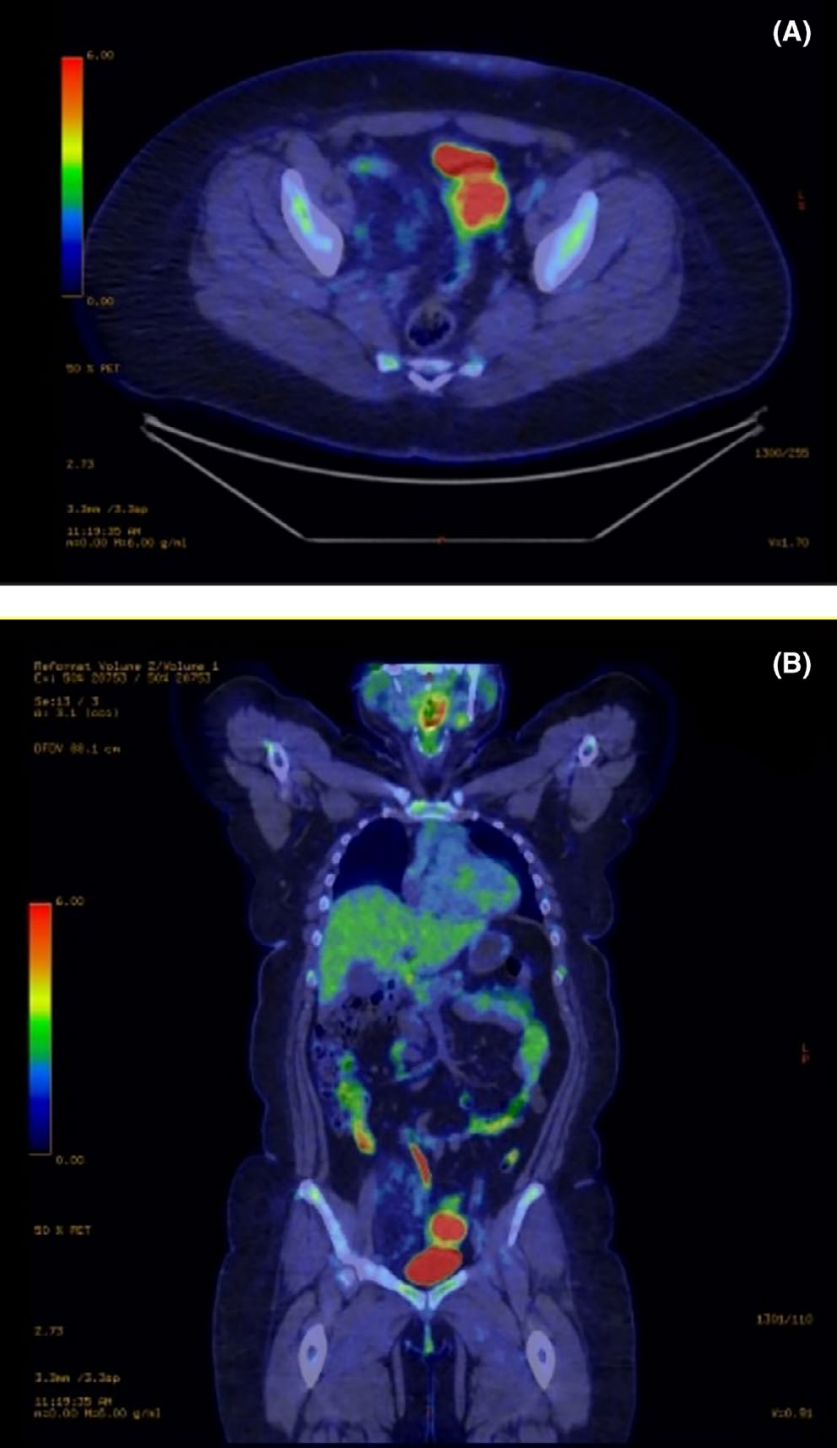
PET CT images showing the right pelvic lipomatous tumor, containing mildly avid soft tissue elements, with no evidence of nodal or metastatic disease. (A) Axial view. (B) Coronal view.
